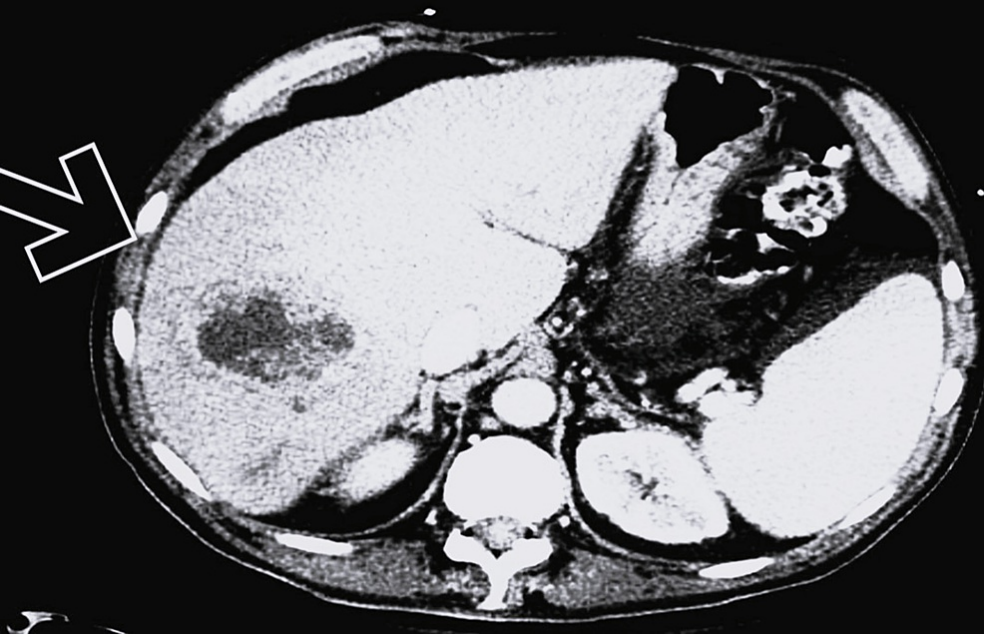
Abdominal computed tomography imaging revealing hepatic abscess, prior to drainage and antibiotics
